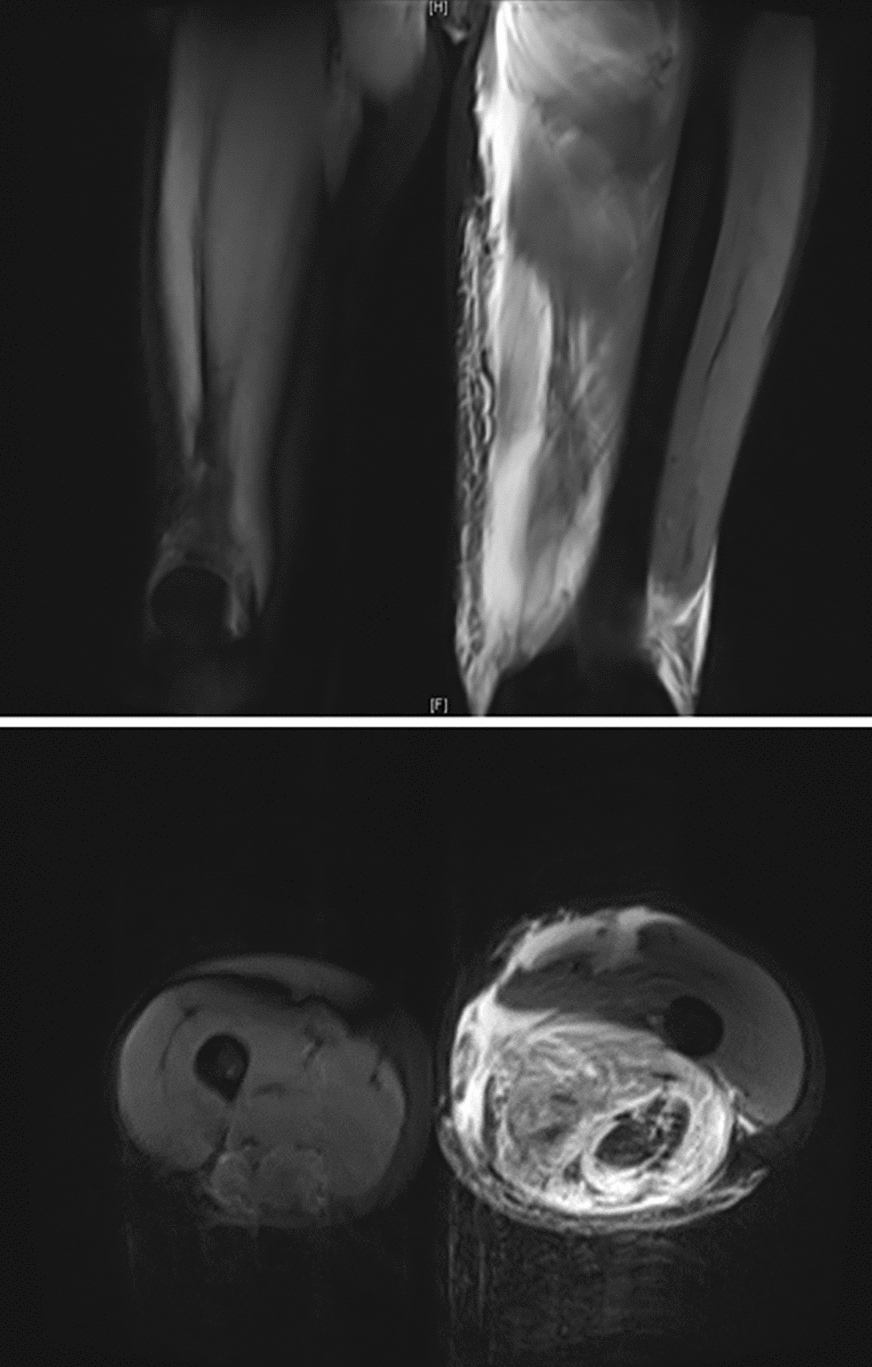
Magnetic resonance imaging (MRI) changes of the left thigh. The posterior and anteromedial muscles of the left thigh (gluteus maximus, rectus femoris, adductor longus, adductor brevis, and adductor magnus) and subcutaneous showed with fat exudative changes, and the interstitial space became blurred
